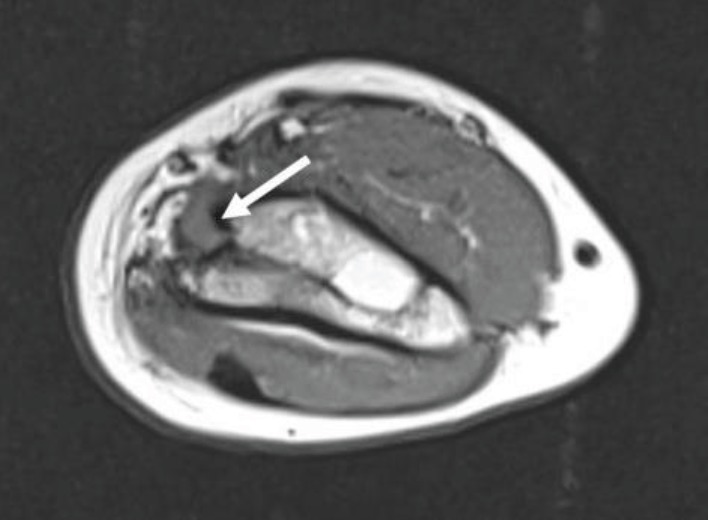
T1 MRI sequence showing median nerve entrapment upstream of the bone callus (white arrow).
